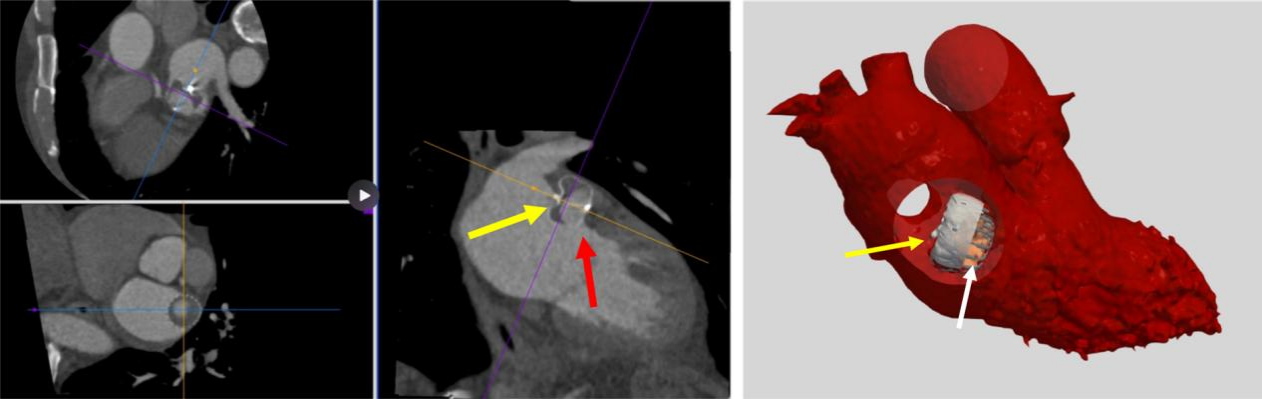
Follow-up CT scan (6 months) of a WATCHMAN FLX device that is not positioned correctly (middle panel rightward pointing arrow) showing a severe leak (middle panel upward pointing arrow). A 3D-segmented model (right panel) demonstrates that the device is rotated by 90°causing the leak at the inferior site of the device. CT, computed tomogram; TOE, transoesophageal echocardiogram.
